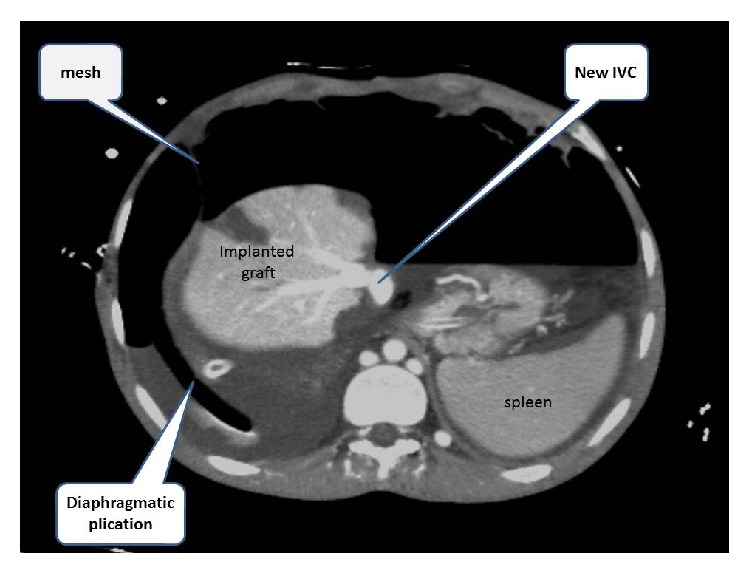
CT view after living donor liver transplantation. Diaphragmatic plication and new IVC are seen.
